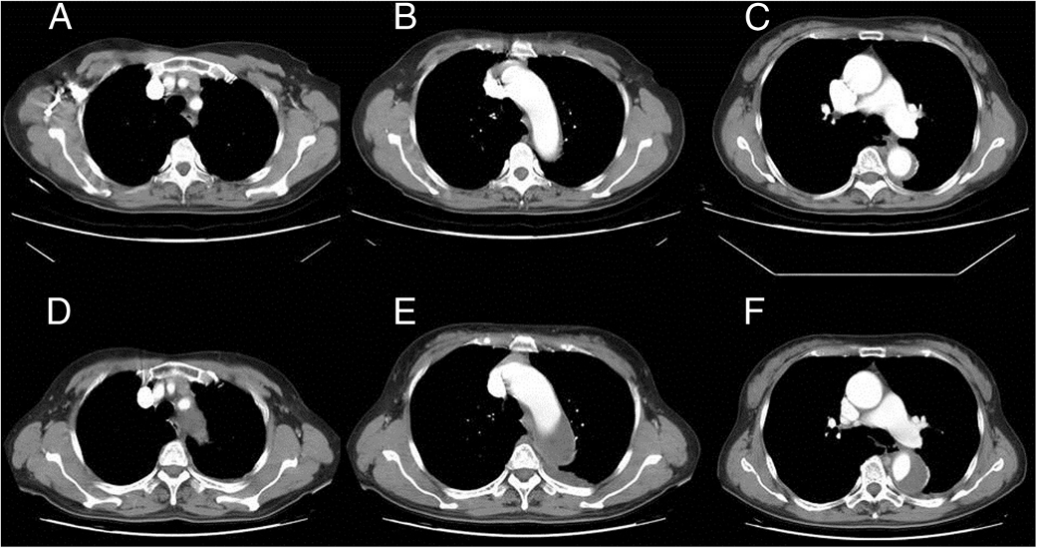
Transverse CT scan showed hematoma formation in the descending aorta. (A-C) One month before admission, descending aortic dissection was diagnosed and the patient was treated with medical therapy. (D-F) At admission, as compared to the prior CT scan an intramural hematoma with false lumen was noted.
